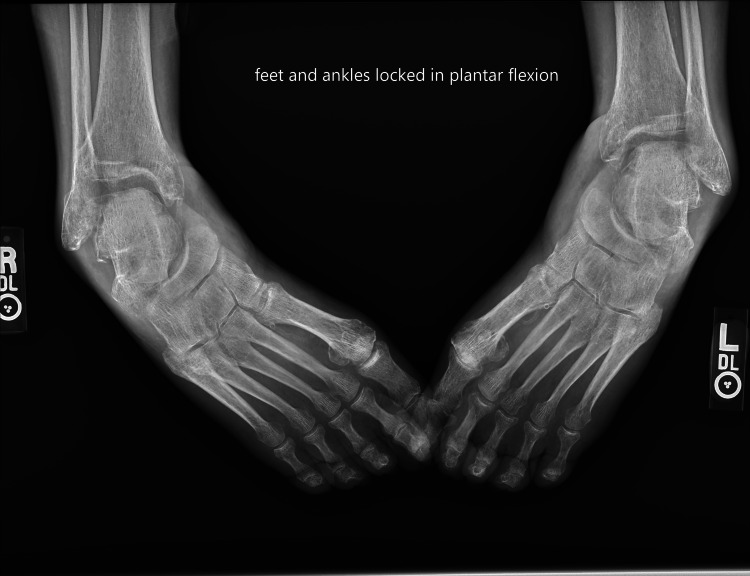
Preoperative AP radiograph of the bilateral ankle and hindfoot Preoperative radiograph demonstrating bilateral hindfoot deformities with overall rigid plantarflexion and adductovarus deformity. AP: anteroposterior
